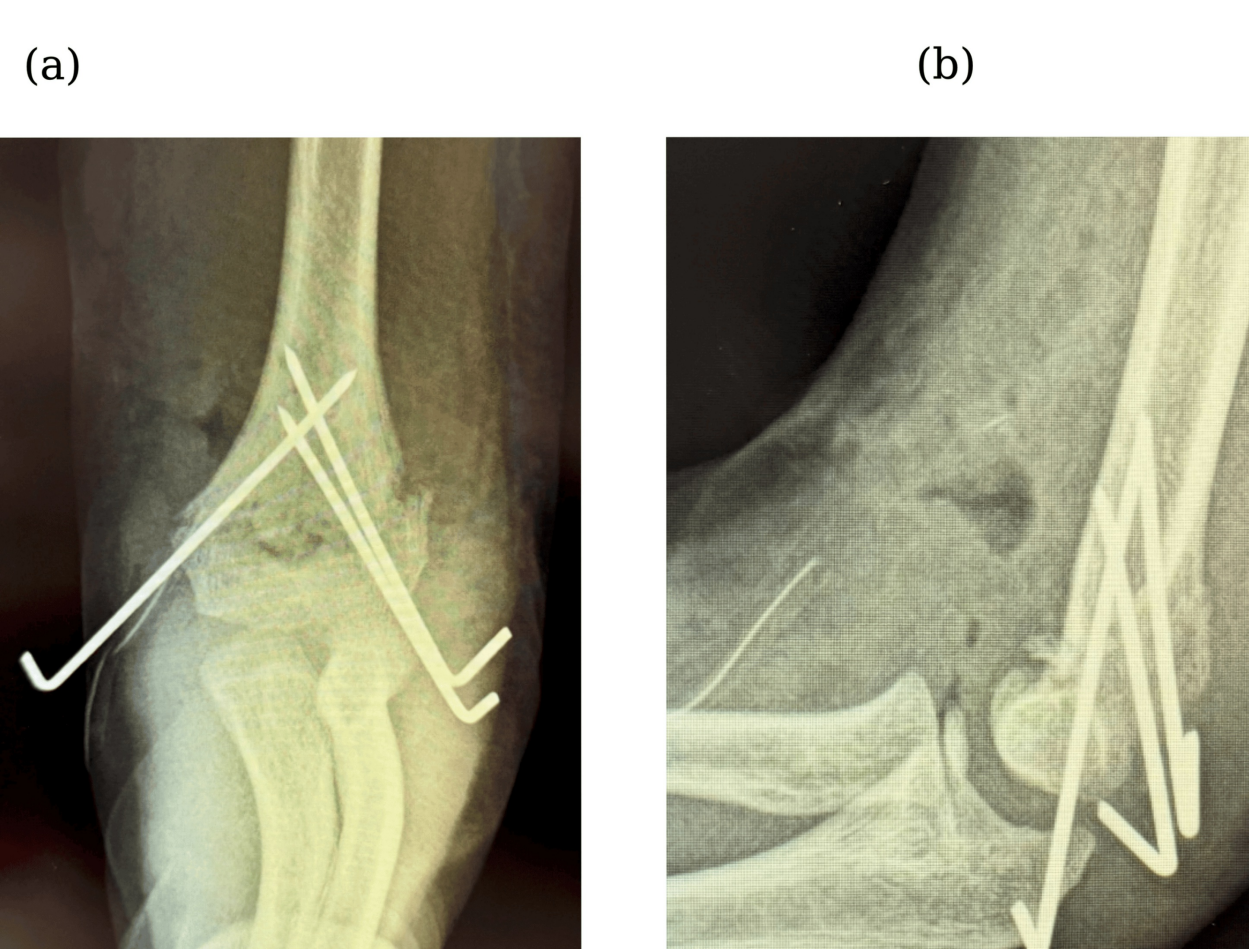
Plain radiograph in (a) frontal view and (b) lateral view taken postoperatively.
